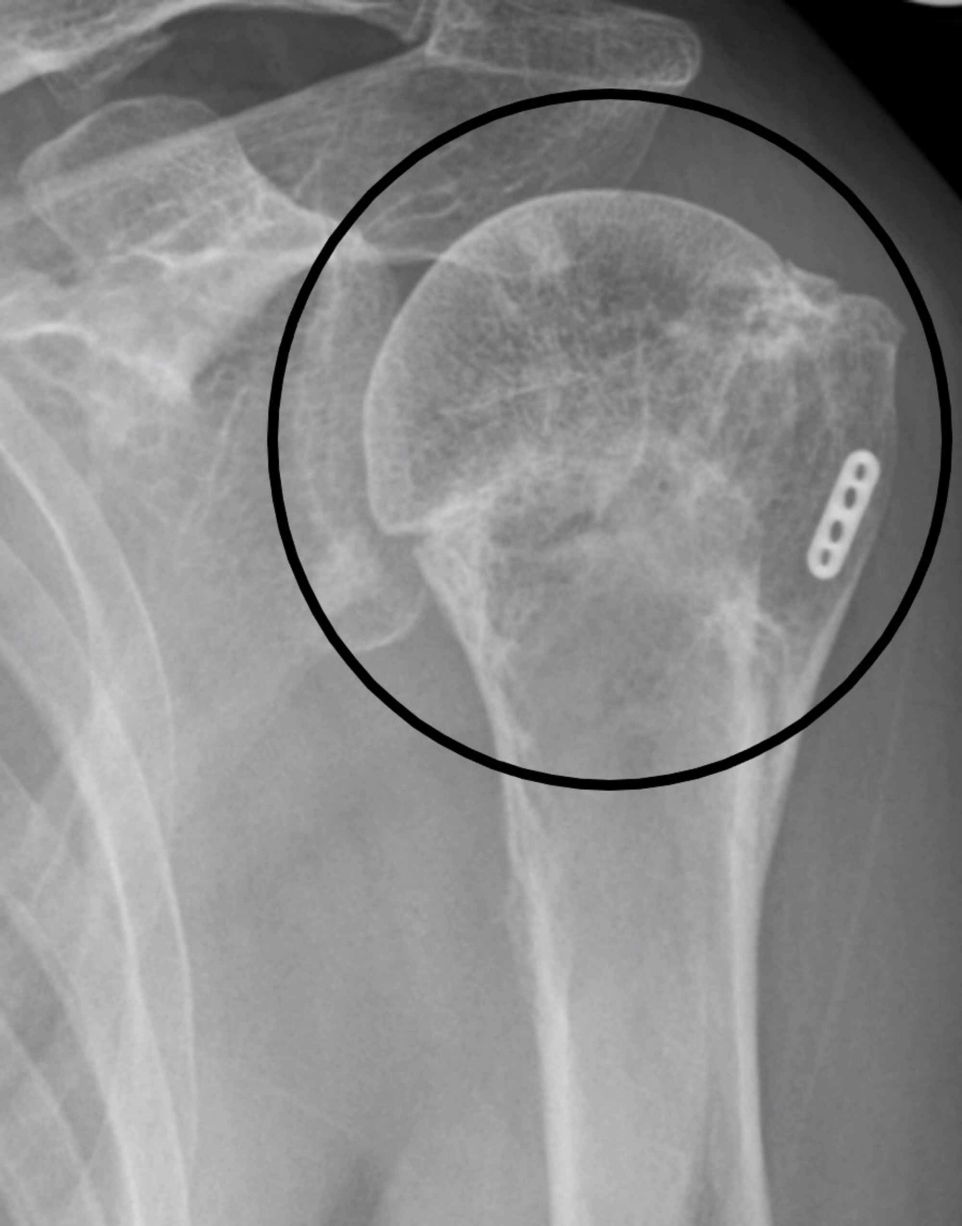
X-ray at three months (encircled healed fracture)
